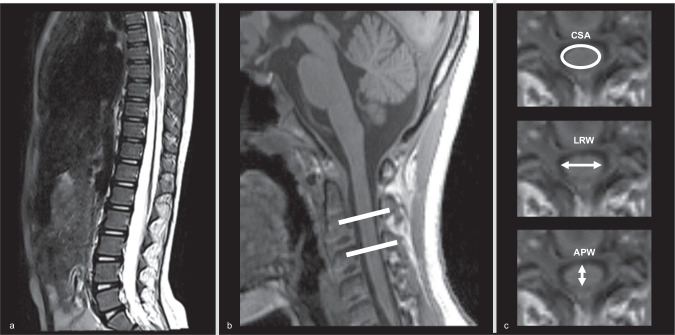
Measurement of morphologic parameters of the cervical spinal cord. a A participant with ID 3 suffered TLSCI due to backbend. A sagittal T2-weighted image taken 14 months post-injury shows atrophy of the spinal cord below the T9 to L1 level. b 3D T1-MPRAGE midsagittal image of the same participant. The white line shows the assessed location of the spinal cord. c Extract the morphologic spinal cord parameters at this location, including spinal cord CSA, APW, and LRW. TLSCI thoracolumbar spinal cord injury, MPRAGE magnetization-prepared rapid gradient-echo, CSA cross-sectional area, APW anterior-posterior width, LRW left-right width.
